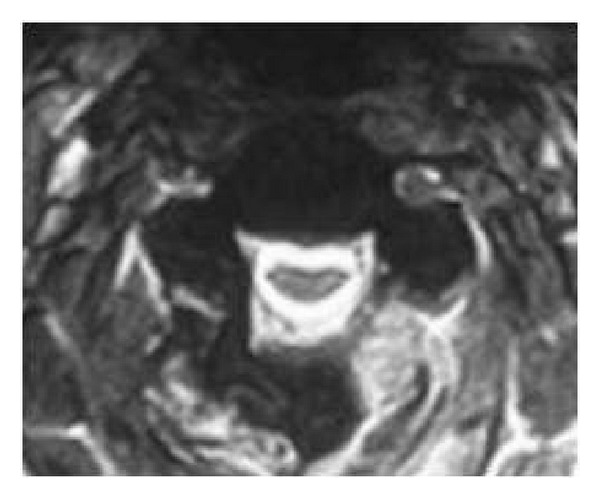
Post-laminoplasty MRI showing the open hinge and space available for the cord created by the decompression.
